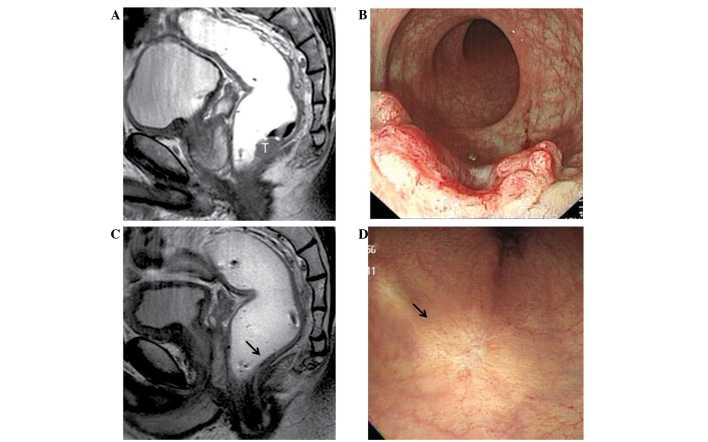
An example of a post-chemoradiotherapy ycCR. (A and B) Pre-treatment tumor (T) in sagittal T2-weighted MRI and endoscopy, respectively. (C and D) Post-chemoradiotherapy sagittal T2-weighted MRI and endoscopy, respectively. (C) Arrow indicates hypointense bowel wall, indicative of fibrosis; (D) arrow indicates whitening of the mucosa. ycCR, clinical complete response; MRI, magnetic resonance imaging.
